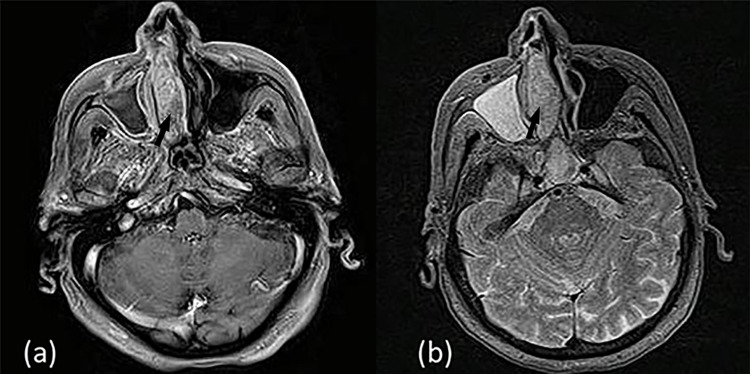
MRI axial section. (a) T1-weighted sequence with gadolinium enhancement (b) T2-weighted sequence.
